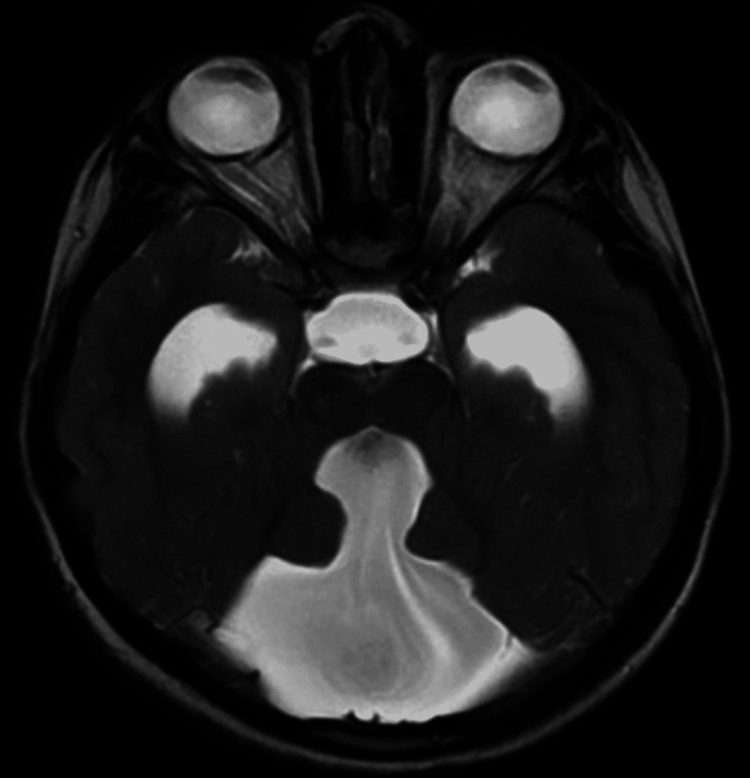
Brain MRI plain axial T2-weighted image shows dilated fourth ventricle communicating with a large posterior fossa cyst.
